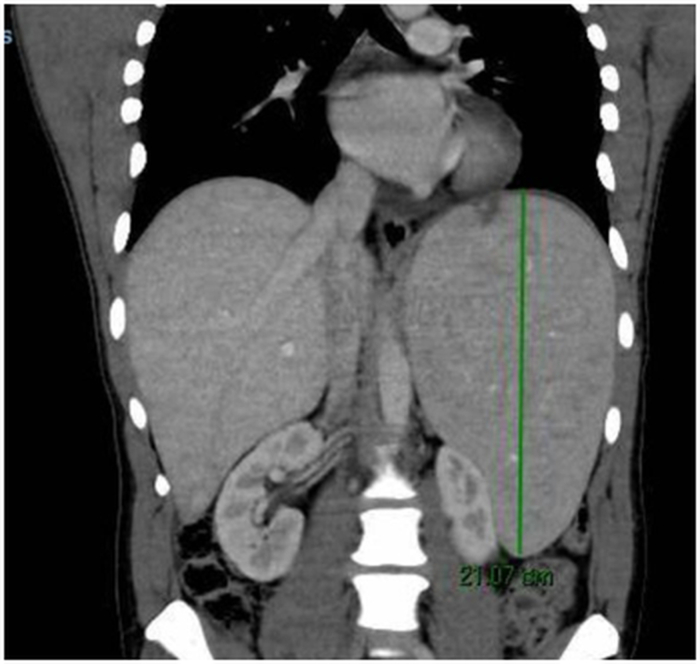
Computed Tomography With Marked Splenomegaly (21.1 cm) and No Focal Splenic Lesions
